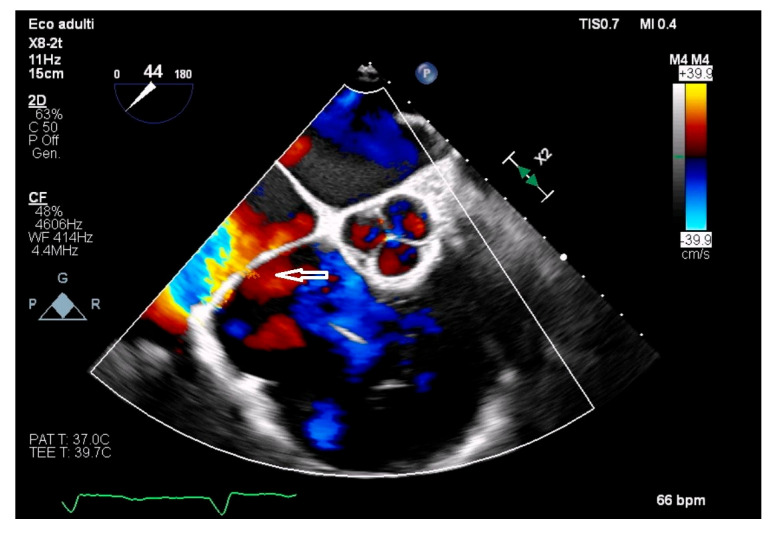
Transoesophageal echocardiogram displaying a membrane subdividing the right atrium into two chambers (cor triatriatum dexter. There is a mild turbulence across the membrane which is represented by a small orifice (white arrow).
